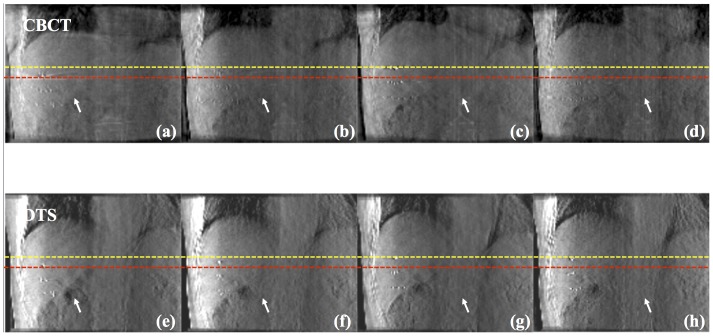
Results (Coronal view) showing 4D CBCT and DTS of liver cancer patient #2 with peak-exhale (a), (e), mid-inhale (b), (f), peak-inhale (c), (g) and mid-exhale (d), (h) phases.
